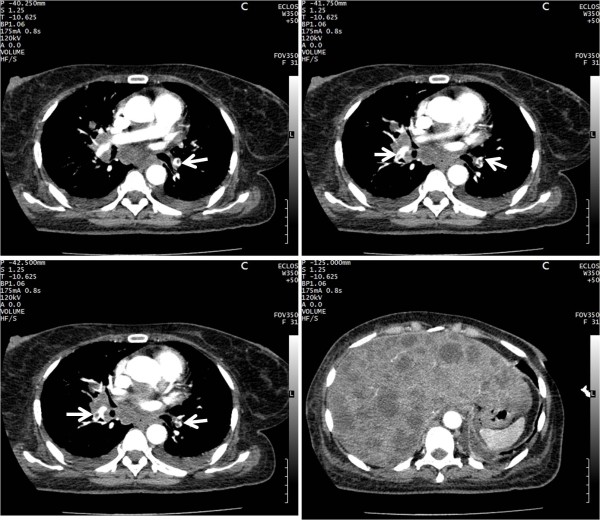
CT angiogram in a 50-year-old woman with sudden and unexplained shortness of breath, who was also restless, shows multiple and bilateral emboli (3a, 3b, 3c, white arrow) associated with multiple enlarged mediastinal and hilar lymph nodes. The liver is enlarged with multiple hypodense and non-enhancing nodules suggestive of metastases (3d).
